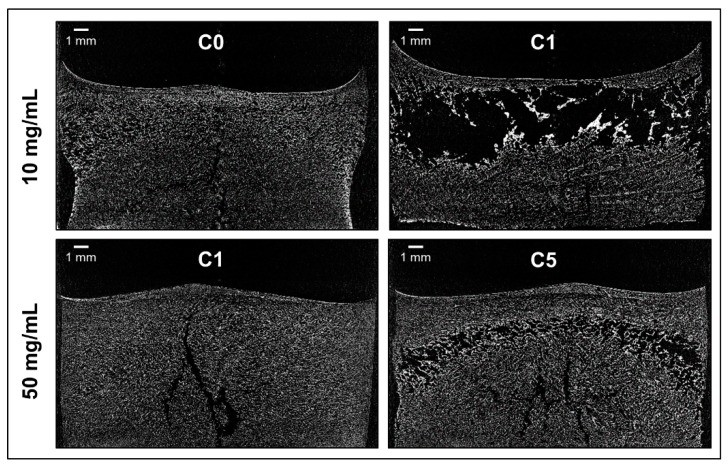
Internal cake structure of F_S_. Representative micro-computed tomography (µ-CT) images for 10 and 50 mg/mL formulations freeze-dried with different lyophilization cycles.
